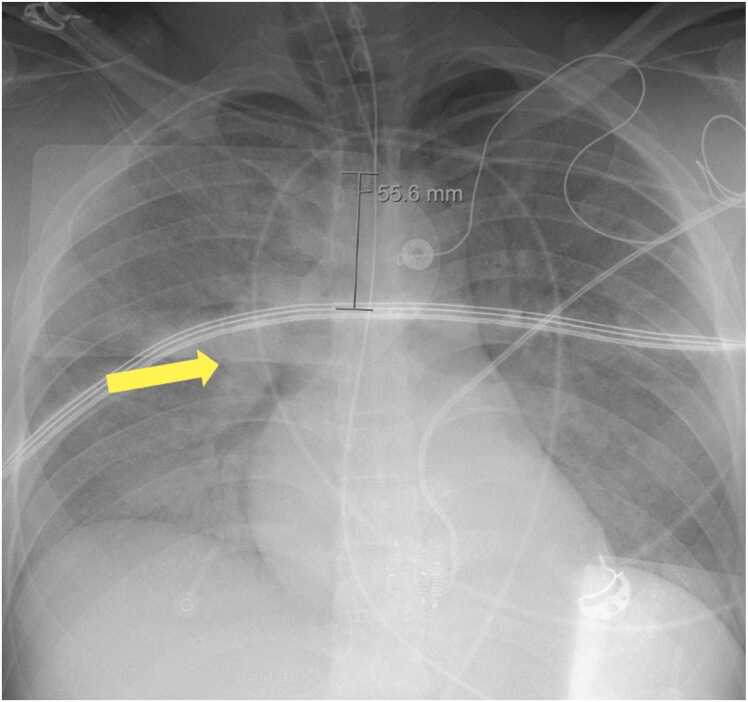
The patient’s chest x-ray on admission showed diffuse opacities throughout the lungs with a consolidation on the right perihilar margin (yellow arrow).
